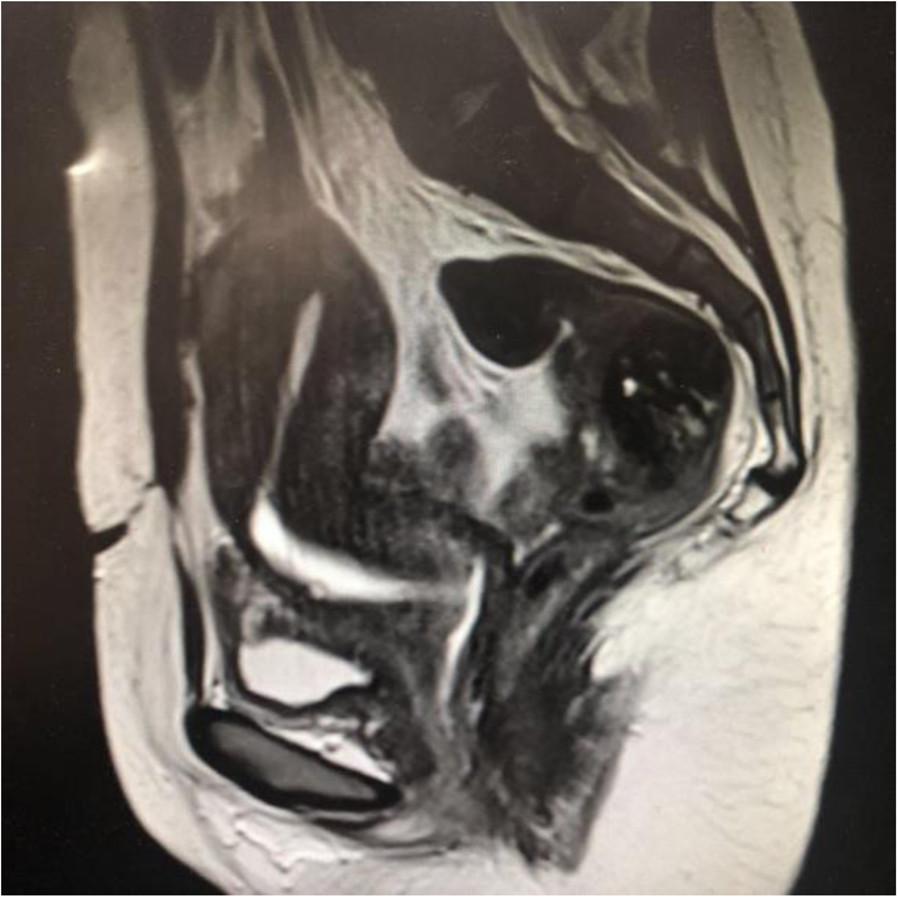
Postoperative picture via MRI
